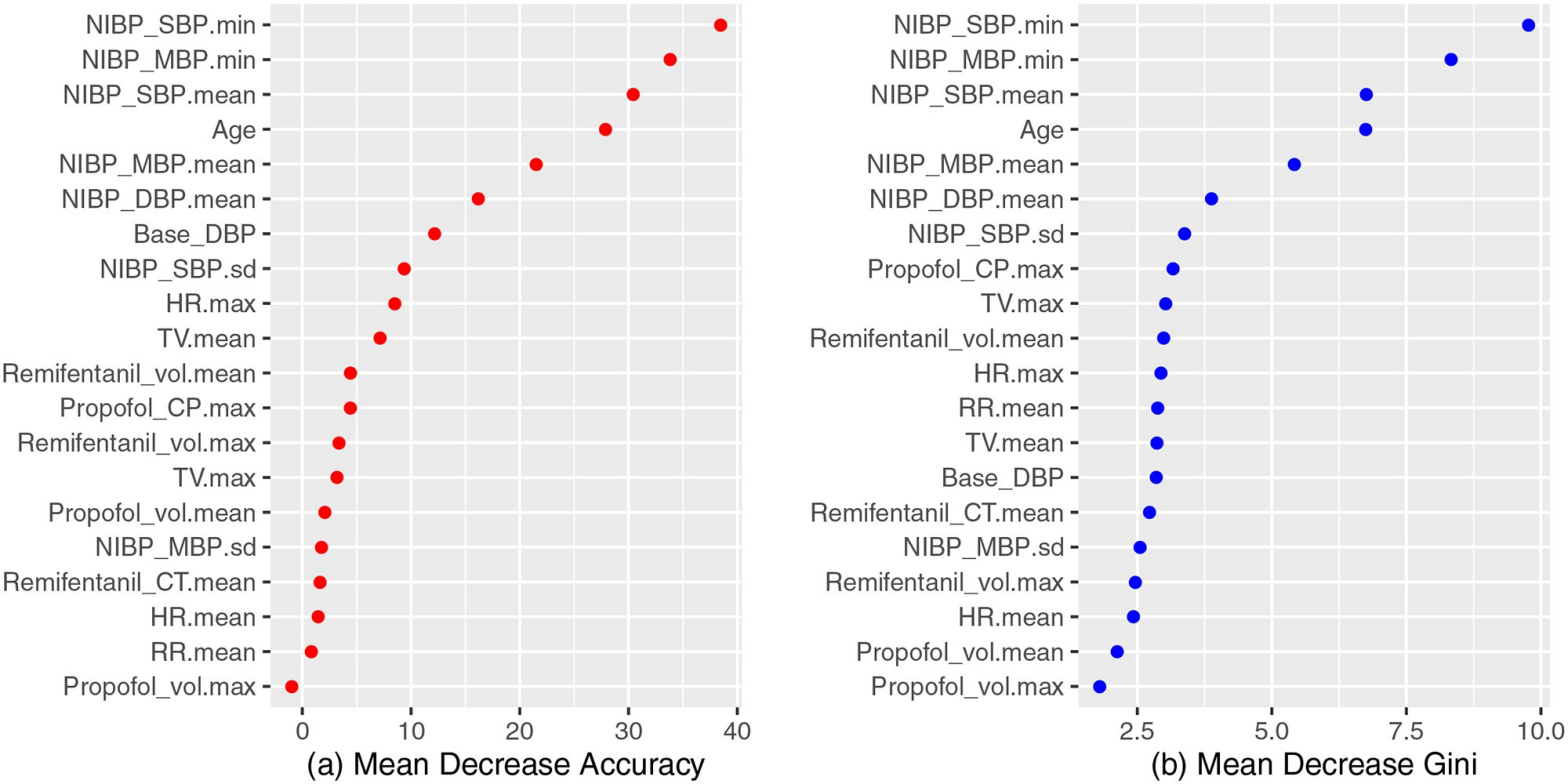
Feature importance plot from the random forest model. NIBP_SBP.min and NIBP_MBP.min were ranked as the first and second most important features based on the importance plot of the random forest model. NIBP, noninvasive blood pressure; SBP, systolic blood pressure; MBP, mean blood pressure; TV, tidal volume; CP, plasma concentration; HR, heart rate; DBP, diastolic blood pressure; RR, respiratory rate; CT, target concentration.
